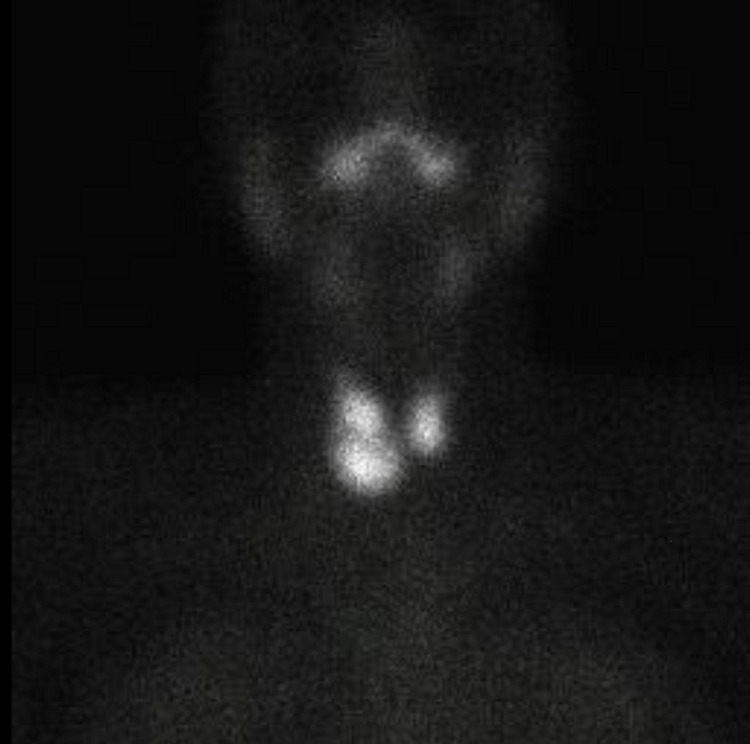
Thyroid scintigraphy Thyroid scintigraphy shows a pattern of uptake corresponding to type 2 MLS.
